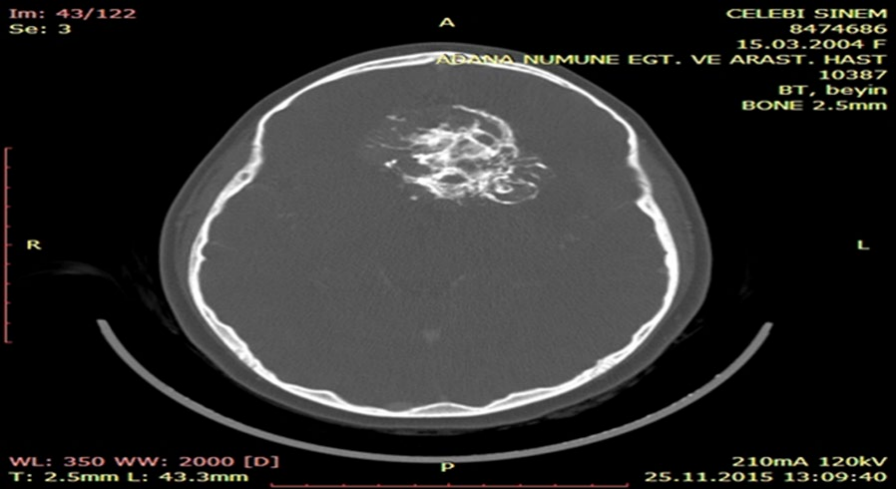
Calcification is clearly detectable in the bone window of the preoperative brain CT image of the patient
